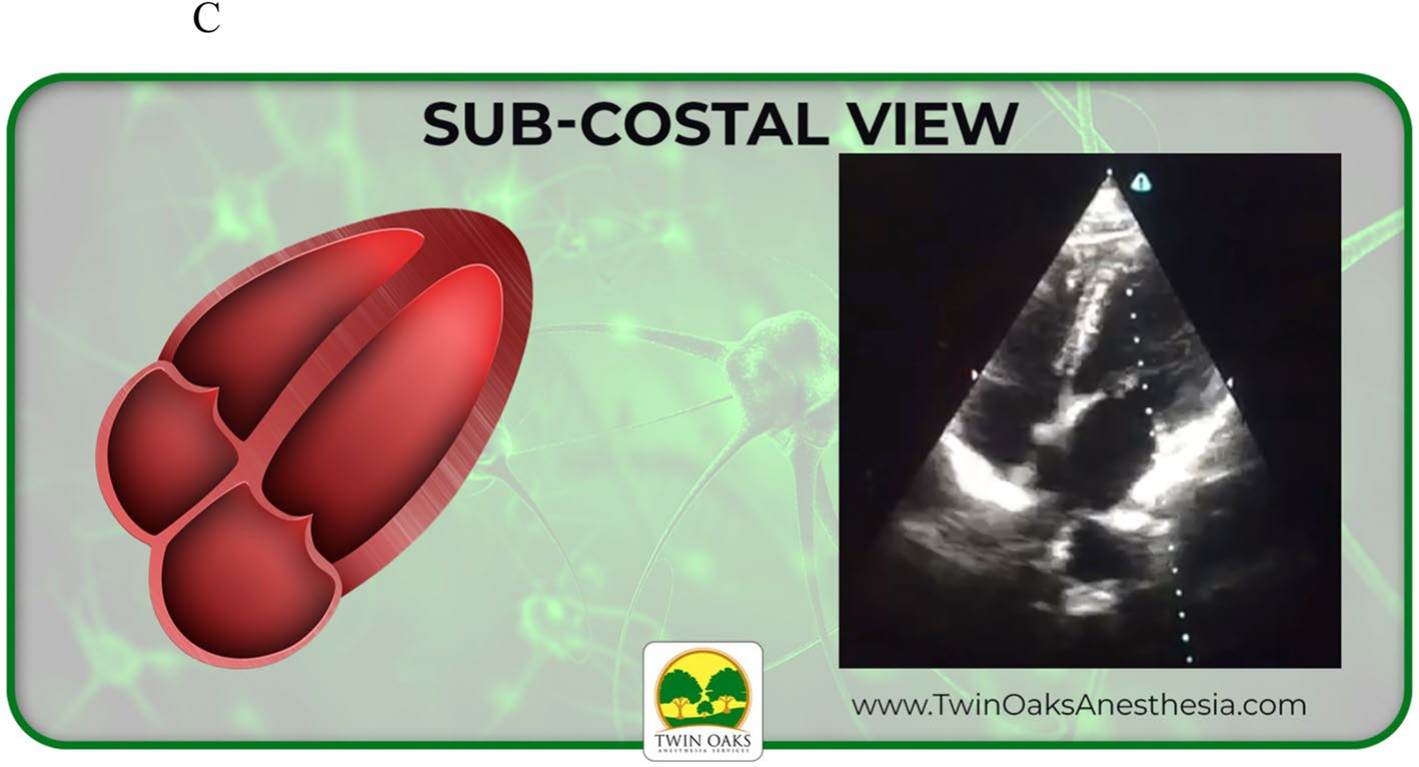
Study graphic and corresponding sonogram of sub-costal TTE view
